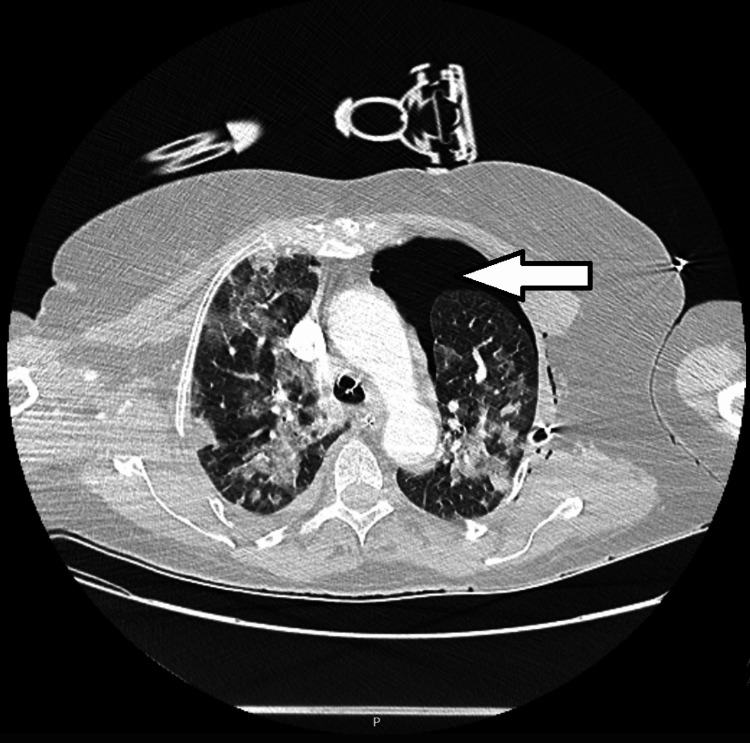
Computed tomographic scan of the thorax on the 10th day in the intensive care unit Left pneumothorax (arrow) and diffuse bilateral subpleural ground glass opacities with fibrotic components are evident.
